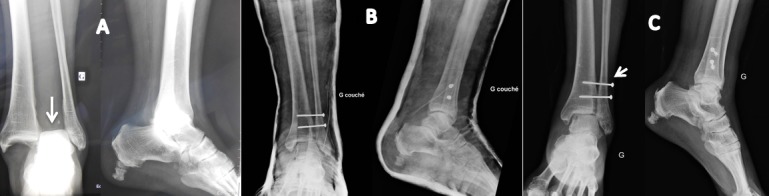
(A) ankle X-ray showed the upward talocrural dislocation (white arrow); (B) X-ray after immediate reduction with two screws and immobilization with cast; (C) ankle X-ray showed the decline off screws (white Arrow)
